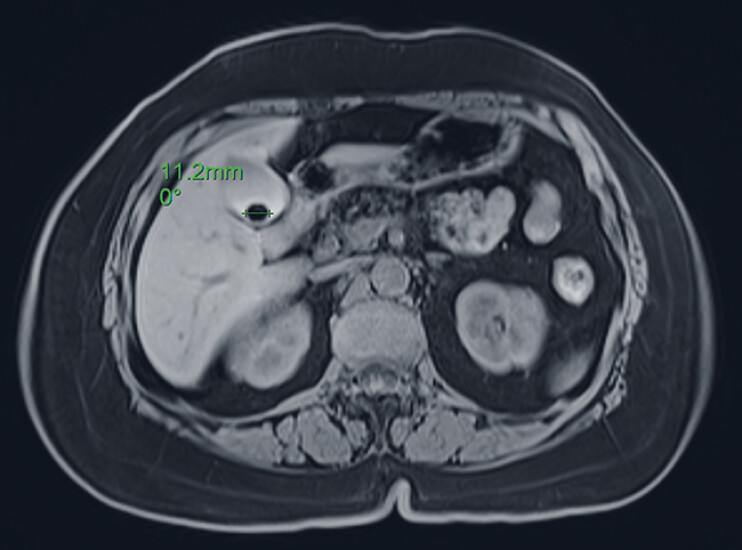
Magnetic resonance imaging of the gallstone.
